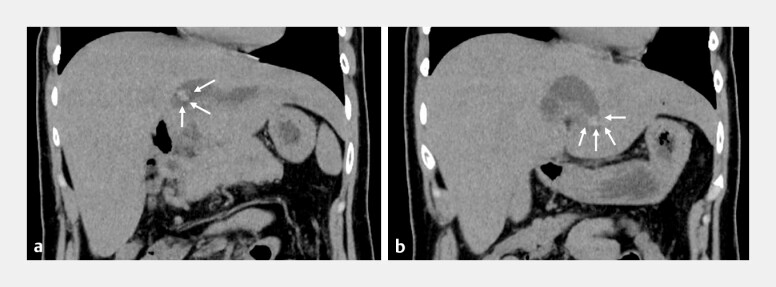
Imaging of computed tomography.
